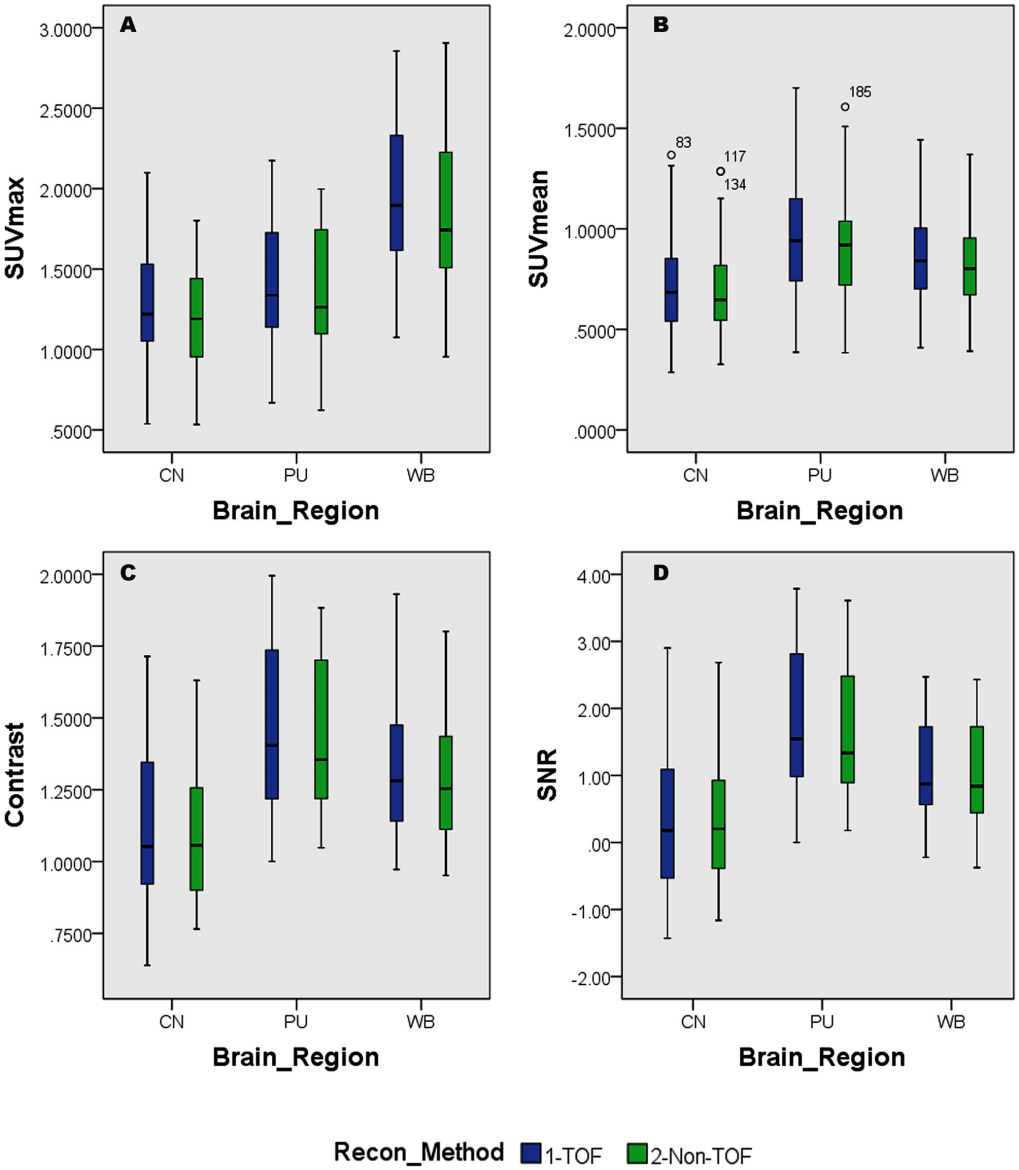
Distribution of the standard uptake values (SUVs) and quantitative parameters with reconstruction methods per each brain regions of ^11^C-PiB brain PET; (A) shows the distribution of individual subject's SUVmax values per segmented brain VOIs; (B) shows the distribution of individual subject's SUVmean values per segmented brain VOIs; (C) shows the distribution of individual subject's SNR values per segmented brain VOIs; (D) shows the distribution of individual subject's Contrast values per segmented brain VOIs; WB, Whole brain; CN, Caudate Nuclei; PU, Putamen.
